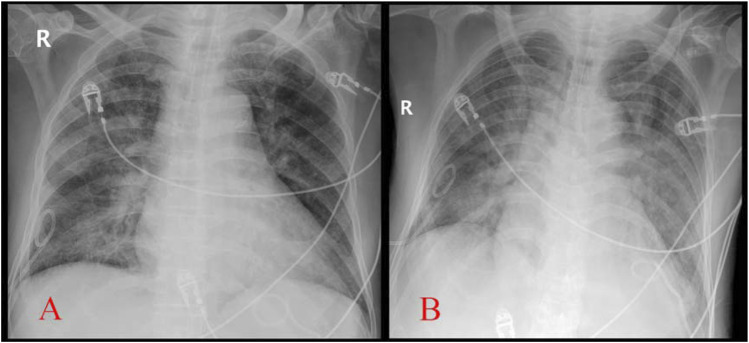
Comparison of chest X-ray before extubation (A, Apr.14) and after reintubation (B, Apr.15) (with permission from the patient.)
